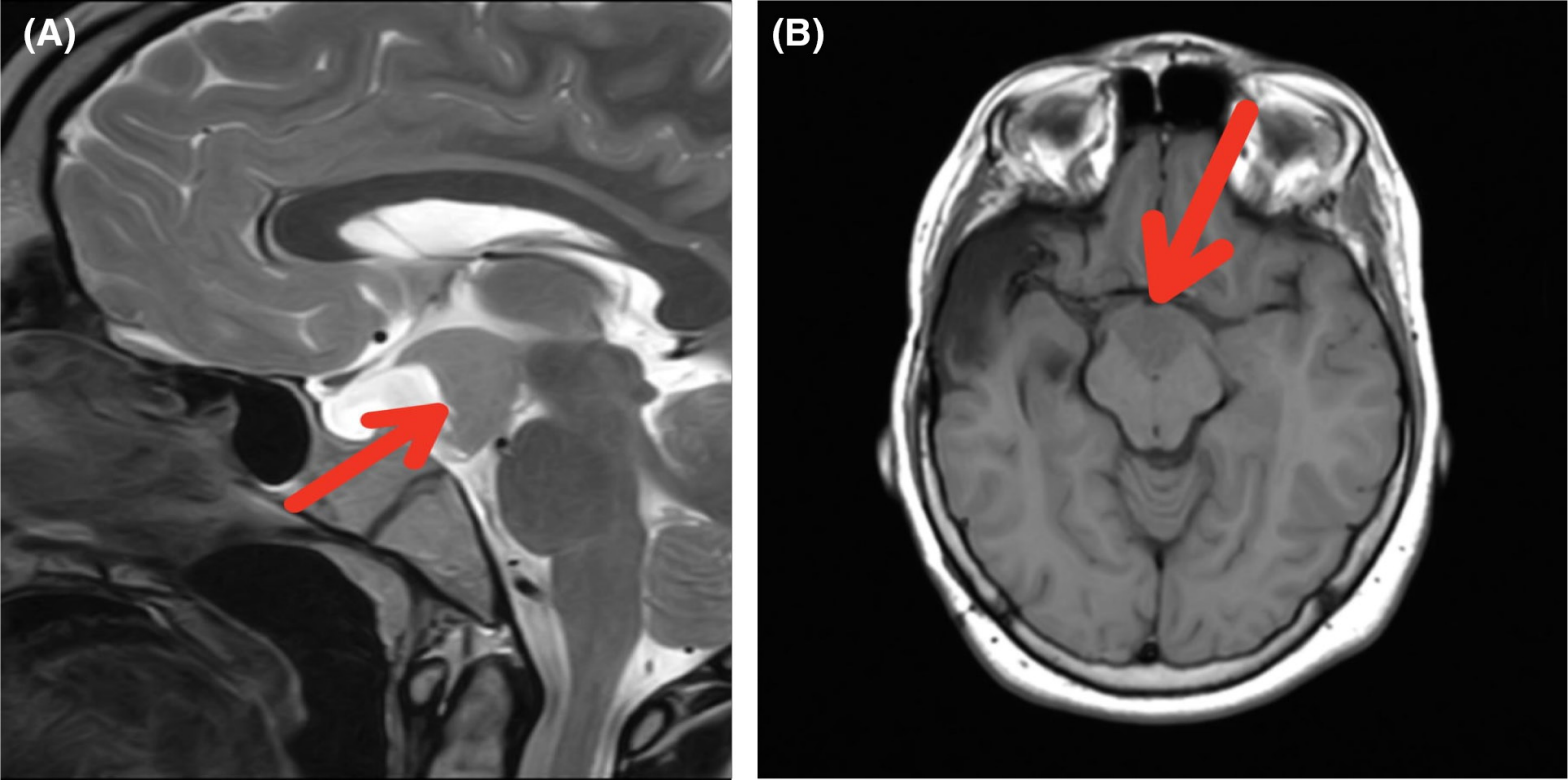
(A) Brain MRI showing a sagittal view of hypothalamic hamartoma; (B) Brain MRI showing an axial view of hypothalamic hamartoma
